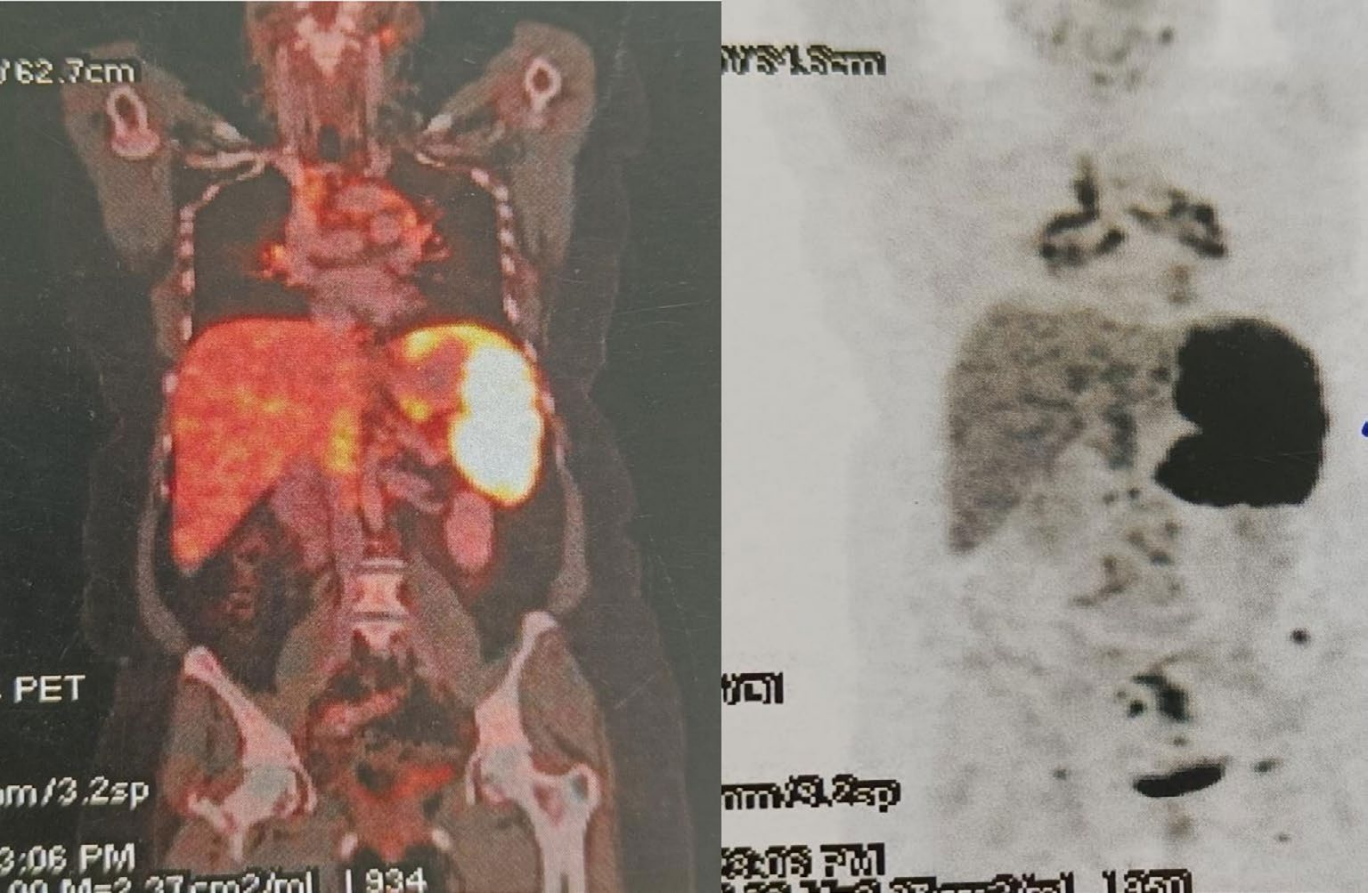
Coronal section on PET‐CT showing increased FDG uptake in liver; spleen; and periportal, perihepatic, para‐aortic lymph nodes.
